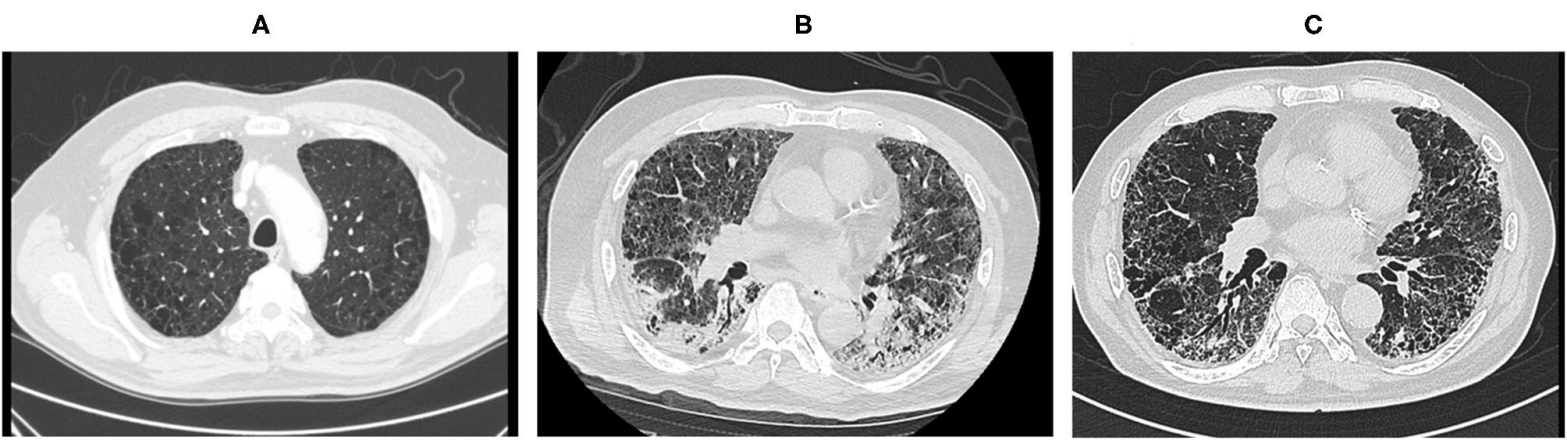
Computed tomography scan before and after hospitalization and before regional hospital transfer. Computed tomography scan images taken (A) 1 week prior to hospitalization, (B) on hospital day 1, and (C) before regional hospital transfer.
